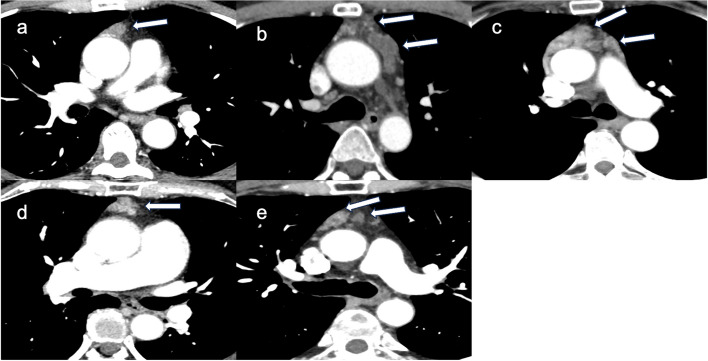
Contrast-enhanced CT scan findings showed the following: Case 1, A 1.2 × 1.2 × 1.7-cm nodule with contrast effect was observed in the anterior mediastinum (a); Case 2, Two cystic lesions of irregular morphology were observed in the anterior mediastinum. They were multifocal without contrast effect (b); Case 3, A small nodular mass with contrast enhancement was observed in the anterior mediastinum (c); Case 4, The size of the irregular mass was 3.0 × 1.2 × 3.5 cm with contrast effect (d); Case 5, Multiple cystic masses and nodules with a diameter of up to approximately 2 cm were found in the anterior mediastinum. The interior did not present with contrast effect, and the tumor margins had contrast effects in the form of rings (e)
